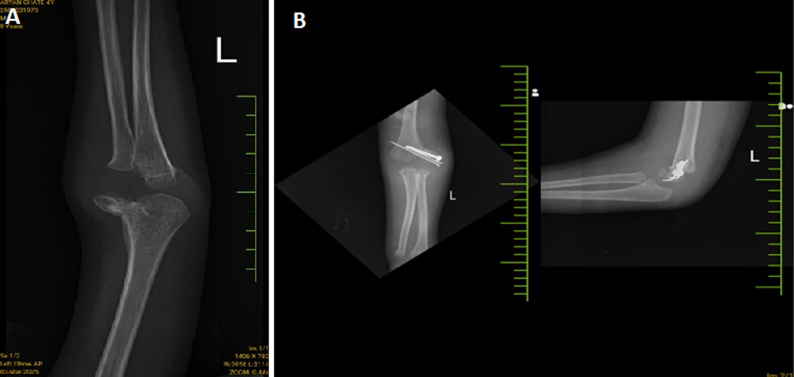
A) X-ray of Salter-Harris type II fracture of the radial head in a 4-year-old child´s left elbow, this type of fracture involves the growth plate and extends into the metaphysis, there appears to be some displacement of the radial head; B) anteroposterior and lateral X-ray views of the left elbow showing a malunited lateral epicondyle fracture surgically treated with osteotomy and K-wire fixation, additionally, a healed supracondylar humerus fracture with internal fixation is noted, heterotopic ossification is present anterior to the elbow joint, which may contribute to ulnar nerve compression
